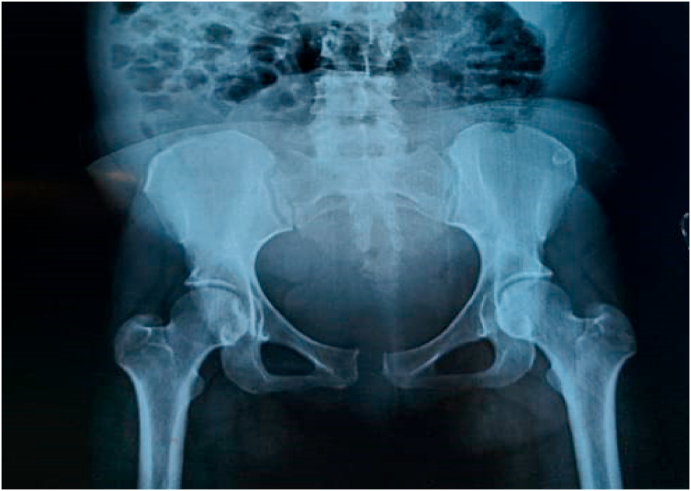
The X-ray of the pelvis showed an enlargement of the pubic symphysis evaluated at 15 mm.
